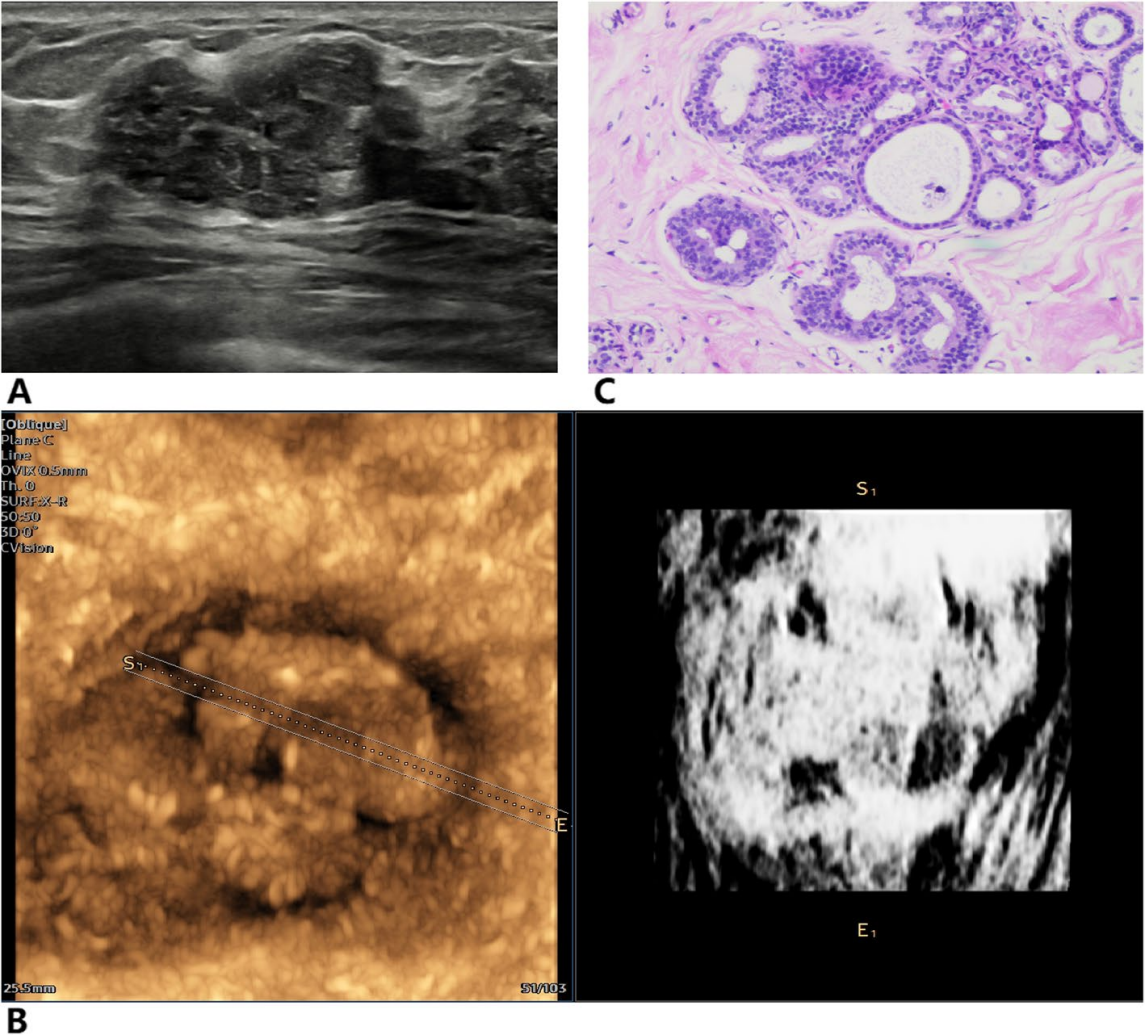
Fibroadenoma of the right breast. A Two-dimensional ultrasound image indicating that the mass was classified as BI-RADS 4A. B Inversion imaging image showing that more than two-thirds of the area within the mass was NTR, the inversion score was 2. C Pathological section showing that the mass was a fibroadenoma
